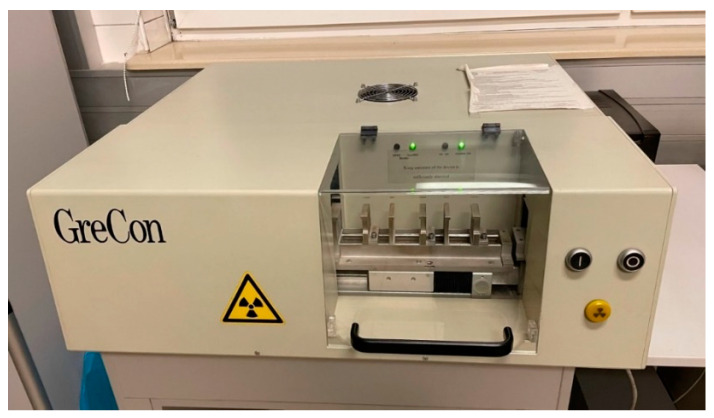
Test stands for the X-ray density profile measured by GreCon.
